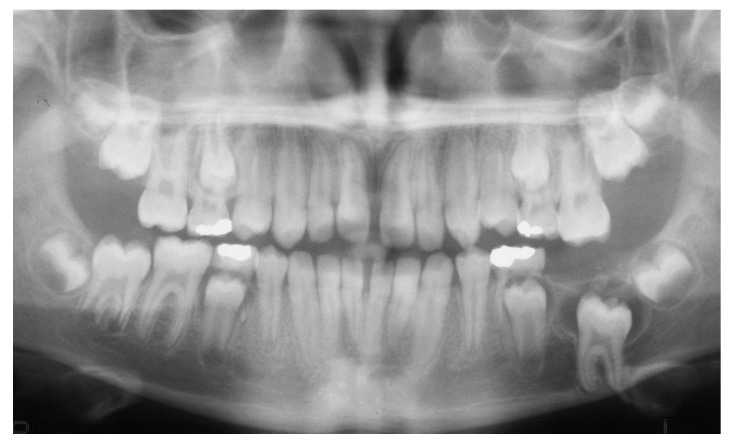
Pretreatment panoramic radiograph showing an impacted mandibular left molar associated with a rather large, well circumscribed, unilocular radiolucent lesion. The root is almost fully developed.
